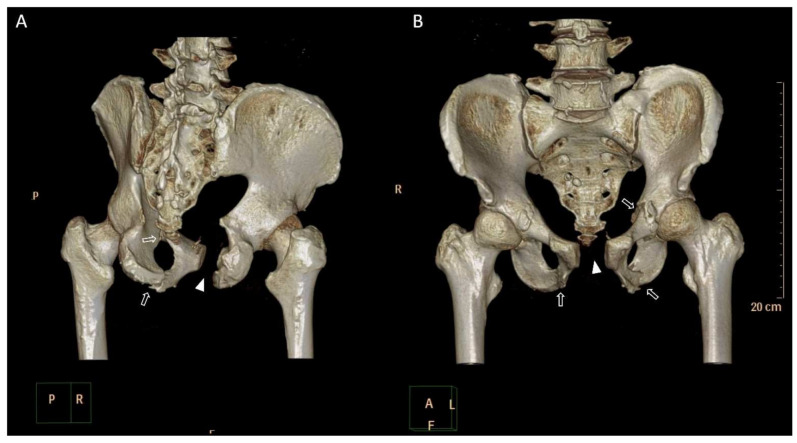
Three-dimensional reconstruction computed tomography scan images showing bilateral superior and inferior pubic ramus fracture (hollow arrows) combined with diastasis of pubic symphysis (triangles) from (A) posterior aspect and (B) anterior aspect.
